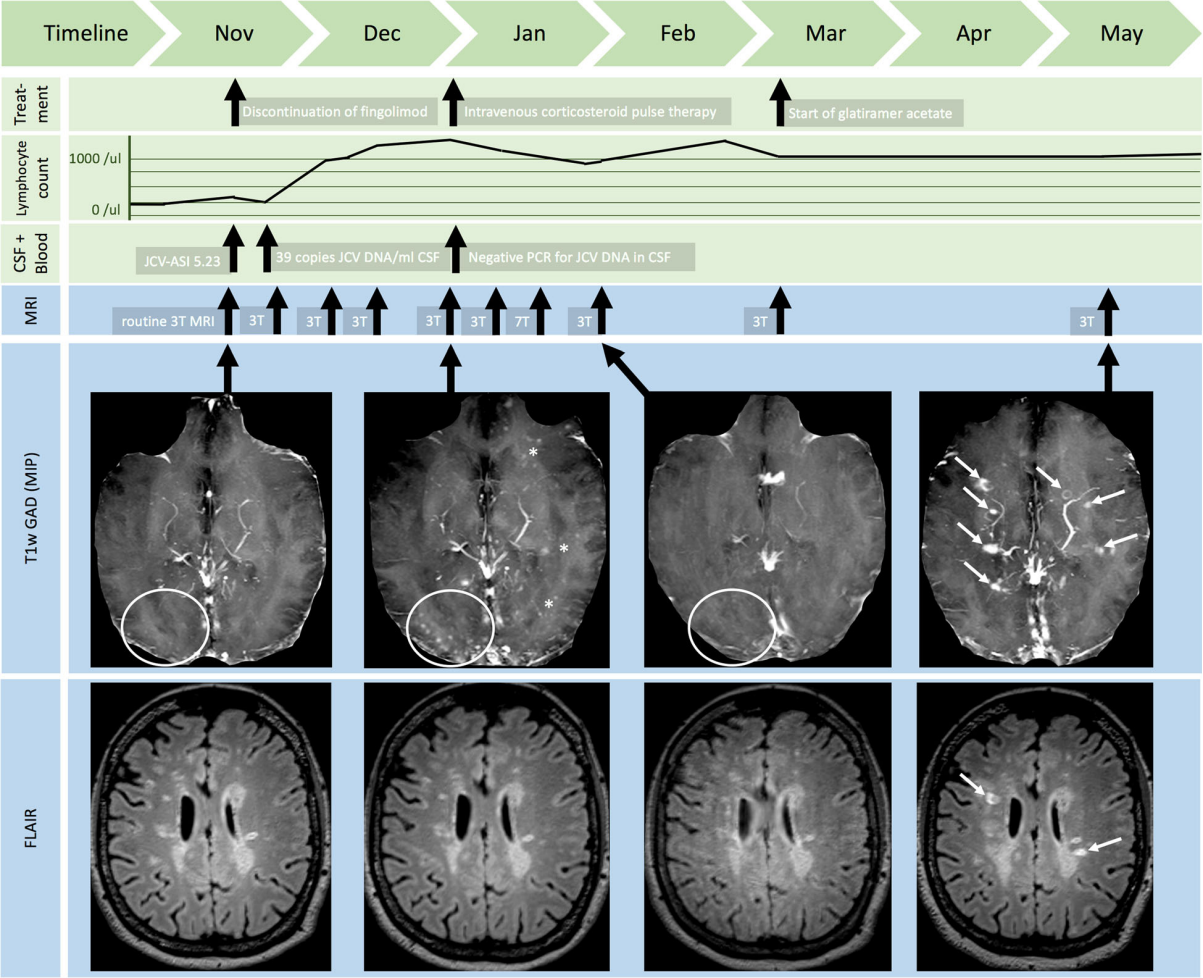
Overview on paraclinical findings and treatment decisions. The figure chronologically displays treatment decisions (first row), lymphocyte counts (second row), laboratory (third row), and MRI findings (fourth and fifth row). At baseline, only very few punctate milky way-like lesions were detectable (circle). After recovery of lymphocytes, new punctate milky way-like lesions developed (circle), and again ceased after corticosteroid pulse therapy (circle). The initial PML lesion in the left hemisphere was stable over time (see Fig. 2). Unfortunately, new MS-like lesions developed after discontinuation of fingolimod (white arrows). Anti-JCV antibody detection and quantification was performed at Unilabs Copenhagen, Denmark. JCV-DNA-PCR from CSF was performed at Institute of Virology, University Clinic of Duesseldorf.
